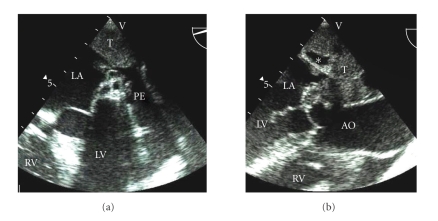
(a) Transesophageal echocardiogram of 4-chamber view showing tumor mass (T) compressing the left atrium (LA). A pericardial effusion (PE) is adjacent to the left ventricular (LV) free wall. RV = right ventricle. (b) Transesophageal echocardiogram of left ventricular (LV) outflow tract in long axis showing homogenous tumor (T) mass with lucent spaces (∗) compressing the left atrium (LA). AO = Ascending aorta. RV = right ventricle.
